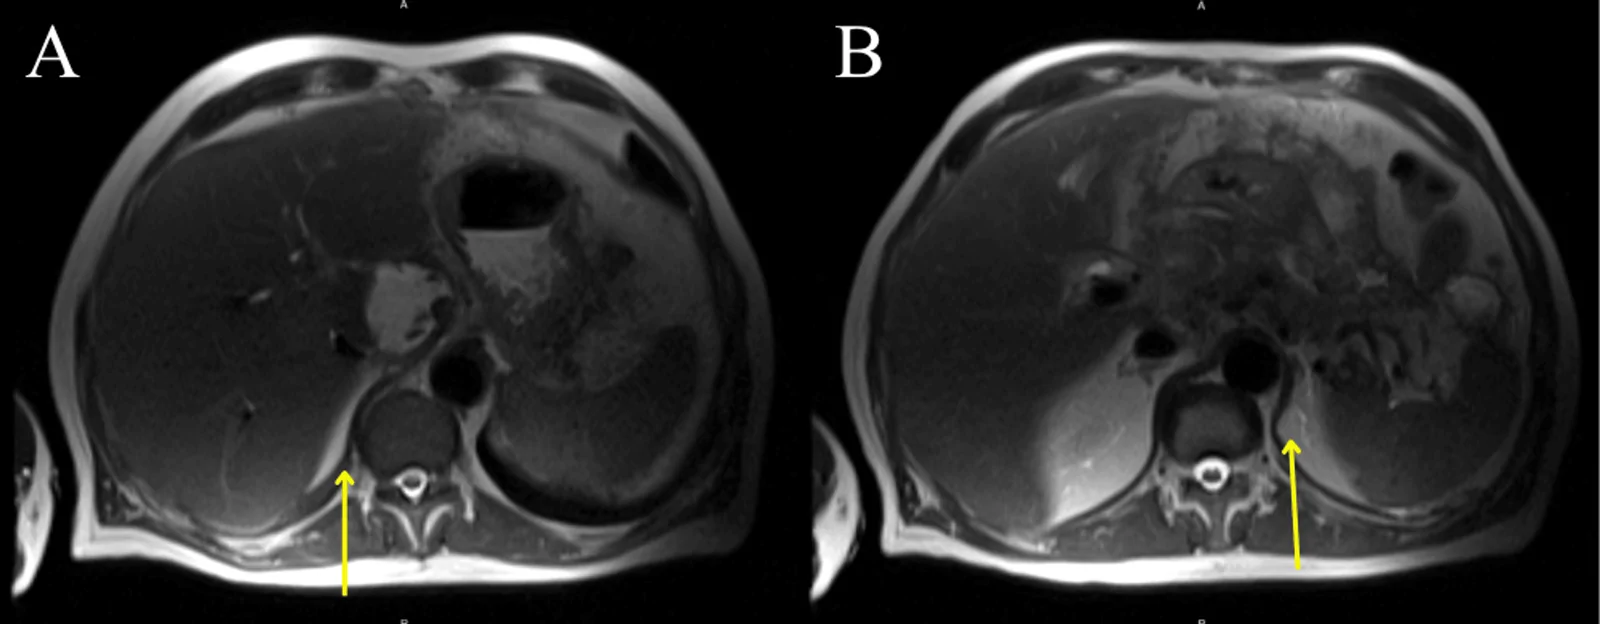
Axial MR images of the upper abdomen without contrast (Panel A) Fluid‑signal collection in the upper abdomen anterior to the vertebral body (arrow), compatible with a small abdominal pseudocyst. (Panel B) Peripancreatic fluid‑signal collection adjacent to the pancreatic tail and splenic hilum (arrow), consistent with a peripancreatic pseudocyst in the setting of pancreatitis.
